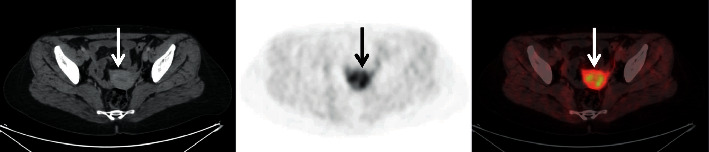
Physiologically intense FAPI-04 uptake was observed in the uterus (arrow) of a 38-year-old female patient without a history of hormonotherapy or oral contraceptive use, with an SUVmax level of 9.76 and an SUVavg level of 5.86.
